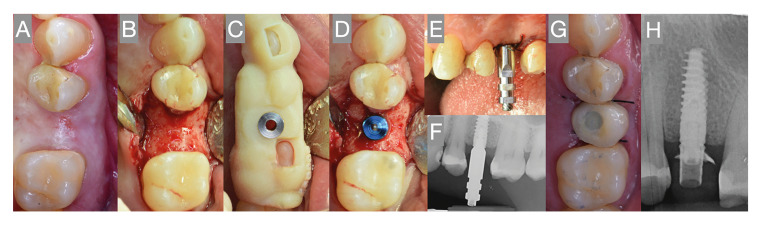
Main steps of the treatment protocol. A: Preoperative image; B: Flap elevation; C: Placement of the surgical guide; D: Drilling sequence and angulation pin; E: Impression transfer coping placement; F: Radiographic assessment before the impression; G: Provisional crown placement; H: Postoperative radiographic assessment.
